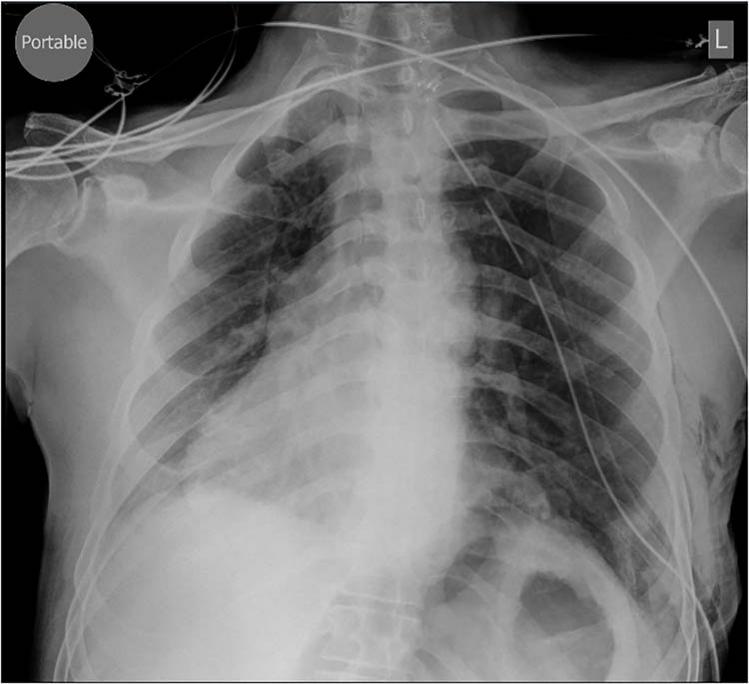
Chest X-ray performed Day 1 postoperatively.
